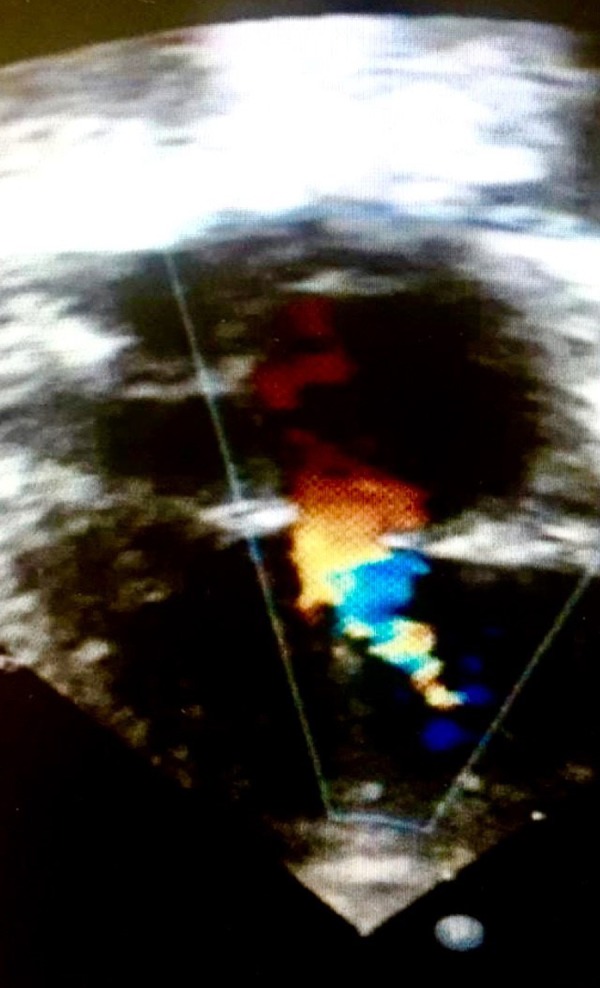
Two-dimensional echocardiography showing a postoperative image of surgical repair of supravalvar mitral ring stenosis. 169 x 300mm.
